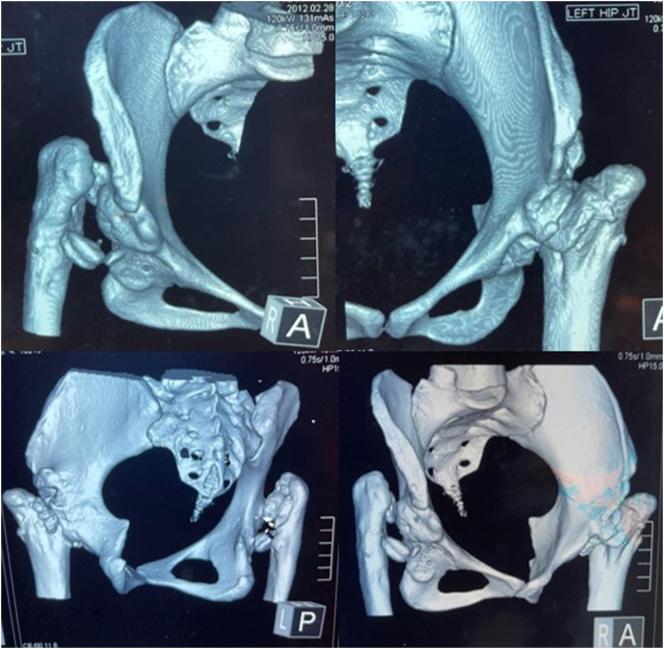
Computed tomography scan showing bilateral destruction of the femoral heads and neck and the acetabula. The left and right femoral canal sizes were 8 mm and 7 mm respectively.
